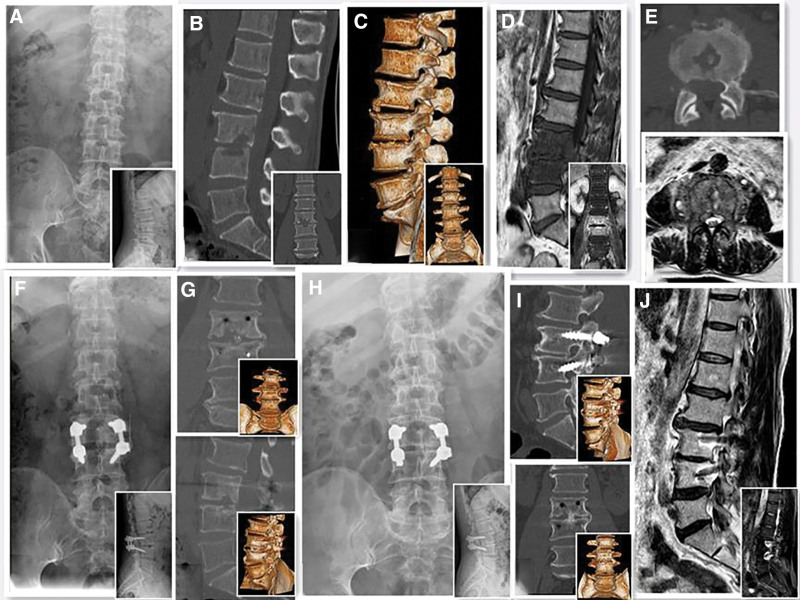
A 54-yr-old man, who was admitted to the hospital mainly because of “low back pain with activity restriction for 3 mo and worsening for 2 mo.” Preoperative diagnosis revealed L3–4 brucellosis spondylitis, thus he was subjected to posterior L3–4 lesion removal, intervertebral bone grafting, and posterior internal fixation. (A) Preoperative X-ray film; (B and C) preoperative CT plain scan + reconstruction; (D and E) preoperative MRI image; (F) X-ray film obtained at 3 mo after surgery; (G) CT plain scan + reconstruction at 3 mo after surgery; (H) postoperative final follow-up anterolateral radiograph; (I) CT plain scan + reconstruction at final follow-up; (J) MRI scan at last follow-up.
